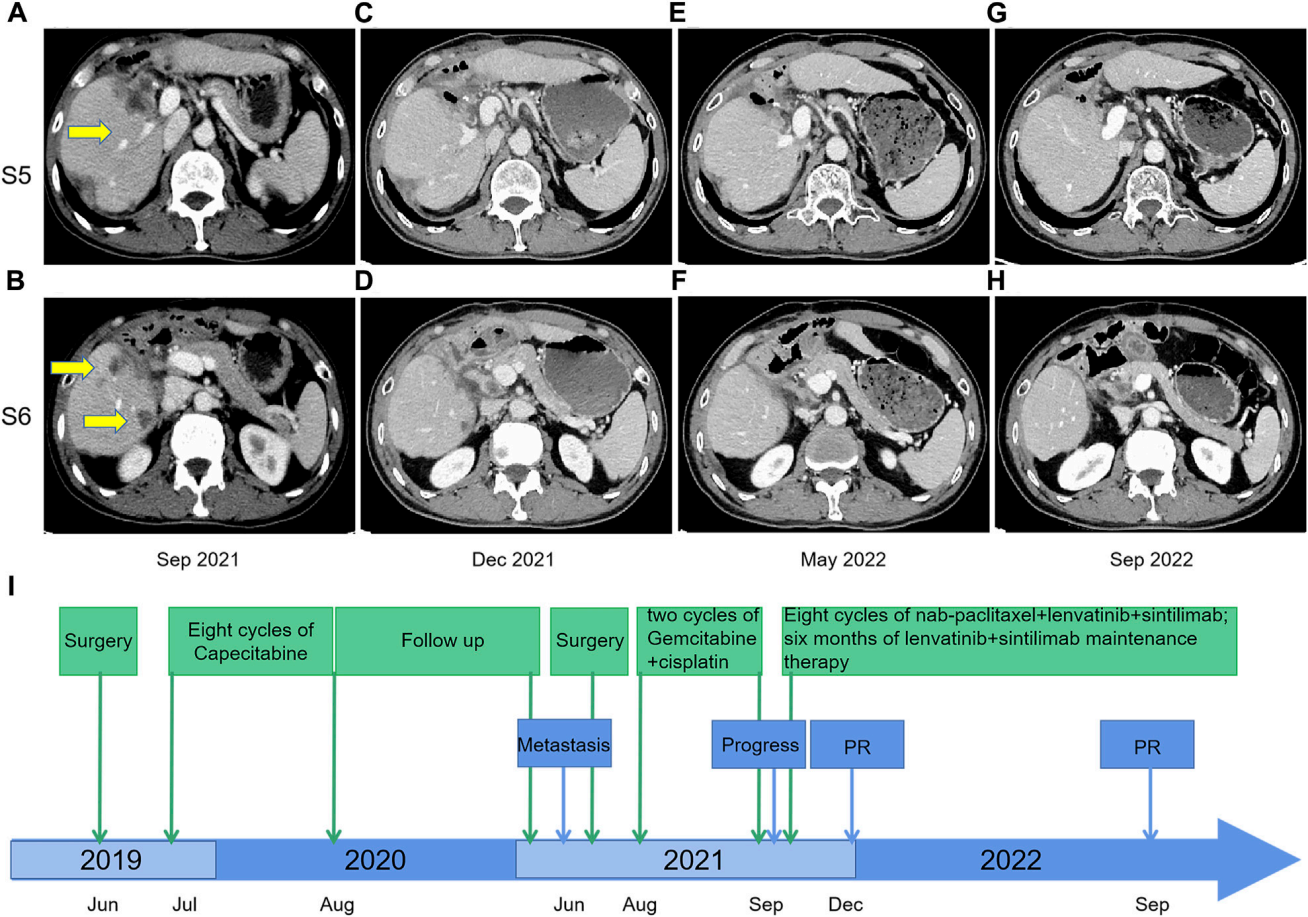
Contrast-enhanced CT scans before and after nab-paclitaxel in combination with lenvatinib and sintilimab treatment; (A,B) September 2021: tumor recurrence and multiple metastases were shown in the liver after two courses of gemcitabine-cisplatin chemotherapy; (C,D) December 2021: response to nab-paclitaxel, lenvatinib, and sintilimab therapy, with size reduction of defined target lesions greater than 30% which corresponds to partial response; (E,F) May 2022: the partial response remained after eight courses of triple therapy; (G,H) September 2022: The CT scan indicated continuing tumor control with maintenance therapy of lenvatinib plus sintilimab from May 2022 to September 2022; (I) Timeline from June 2019–December 2022.
